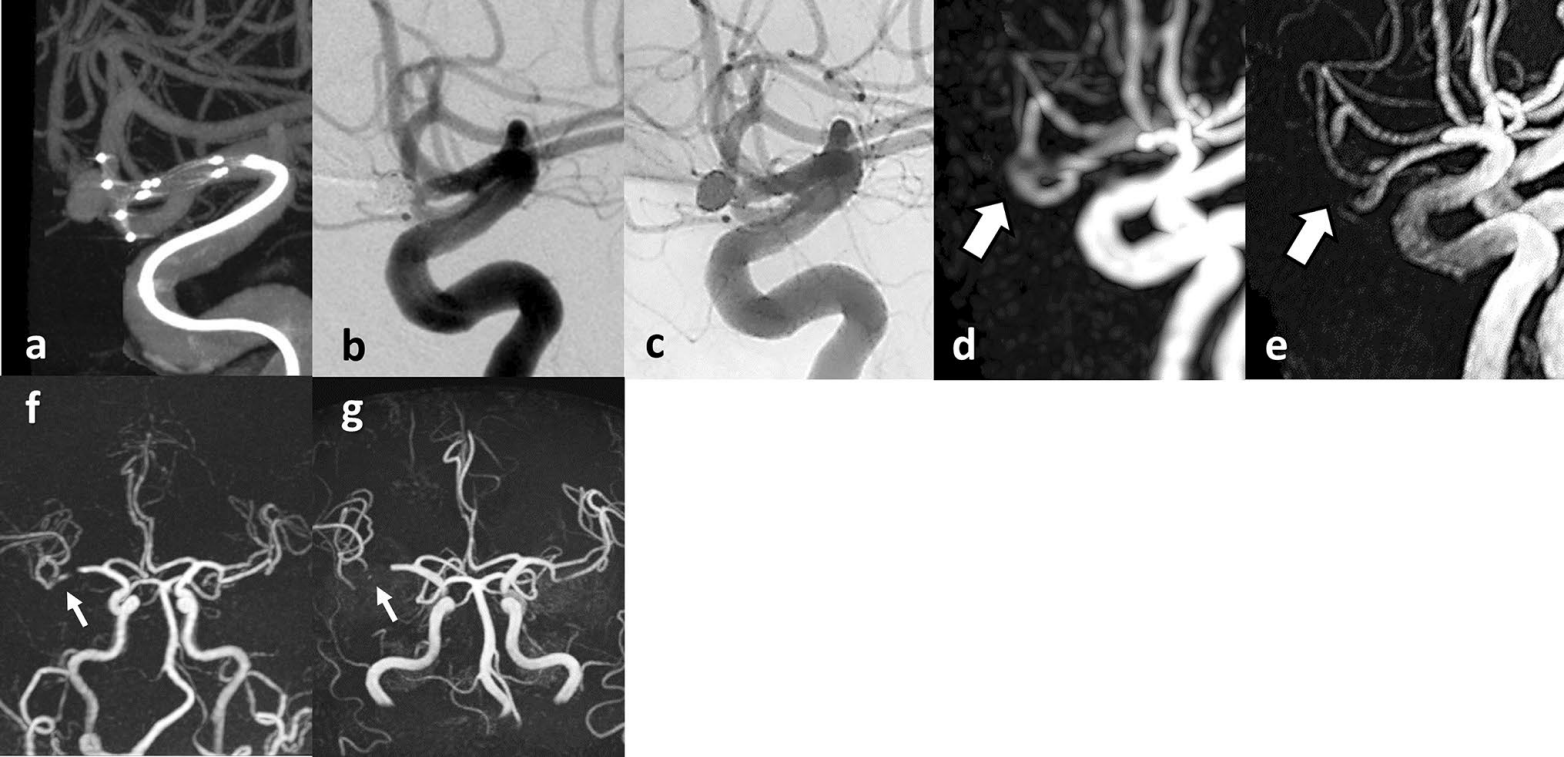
A 73-year-old male patient with an unruptured right MCA bifurcation aneurysm (Case 5). a CBCT after PulseRider placement (T shape, 8 mm, 2.7–3.5 mm). b Postoperative DSA image after coil embolization with PulseRider. c Postoperative native DSA image after coil embolization with PulseRider. d Silent MRA image shows the area signal at the aneurysmal neck and parent artery in the bifurcation (white arrow). e TOF-MRA failed to depict the area signal shown by the PulseRider device (white arrow). f The entire image of silent MRA. The signal around the aneurysmal neck was visualized (white arrow). g The entire image of TOF-MRA (anteroposterior view). TOF-MRA failed to demonstrate the total area signal at the PulseRider placement location (white arrow). *MCA* middle cerebral artery, *CBCT* cone-beam computed tomography, *DSA* digital subtraction angiography, *MRA* magnetic resonance angiography, *TOF* time-of-flight
